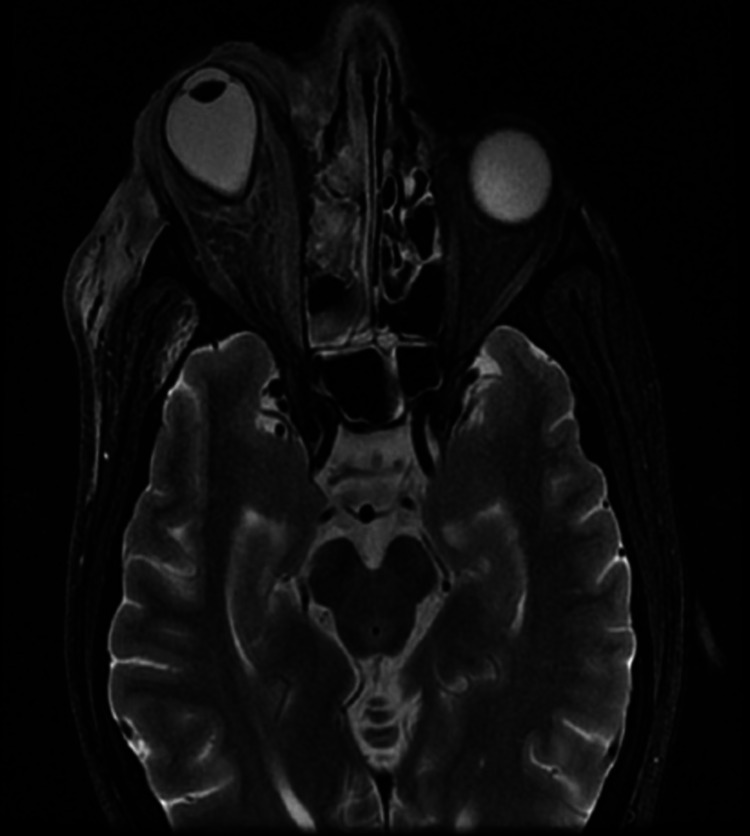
MRI T2 brain axial view The image shows right orbital cellulitis and sinusitis (indicated by proptosis, enhancement of soft tissue, and sinuses) MRI: magnetic resonance imaging
